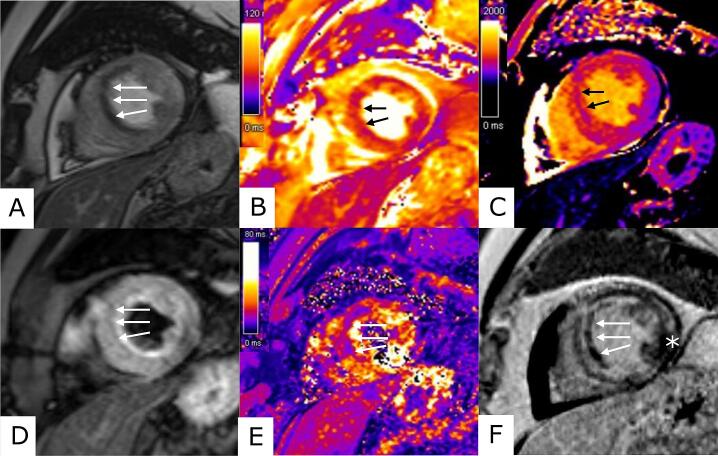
CMR images of intramyocardial haemorrhage. CMR images of a patient with an acute anteroseptal infarction with IMH. Cine showing anteroseptal akinesia, indicated by the white arrows (A). Black arrows indicate IMH in the T2 map and T1 map (B, C). White arrows indicate IMH as a hypointense core within a hyperintense area in the T2W STIR and T2* map (D, E). LGE demonstrating MVO, indicated by the white arrows. The white Asterix shows normal myocardium (F). CMR: cardiac magnetic resonance, IMH: intramyocardial haemorrhage, T2W STIR: T2-weighted short-tau inversion recovery, LGE: late gadolinium enhancement, MVO: microvascular obstruction.
